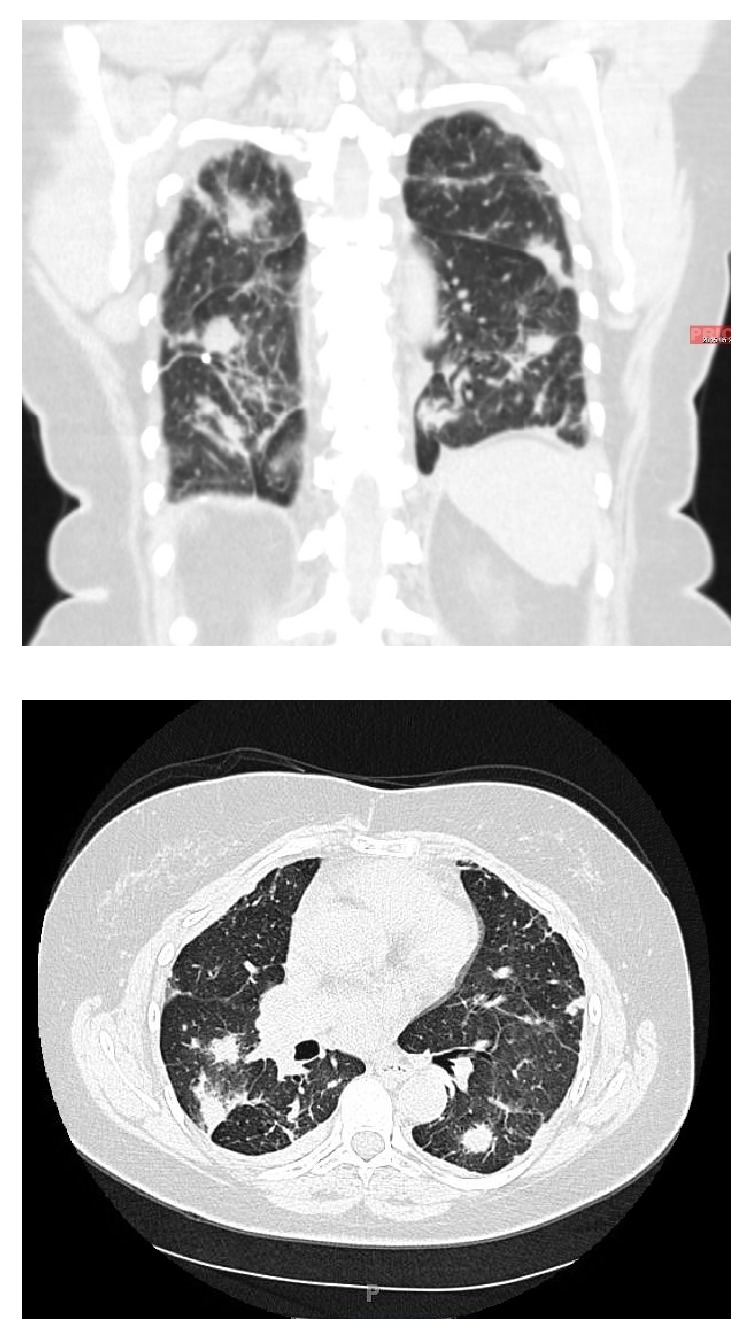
Computed tomography (CT) scan of the chest reveals diffuse parenchymal nodularity, with no honeycombing, hilar, or mediastinal adenopathy, consistent with stage III sarcoidosis.
